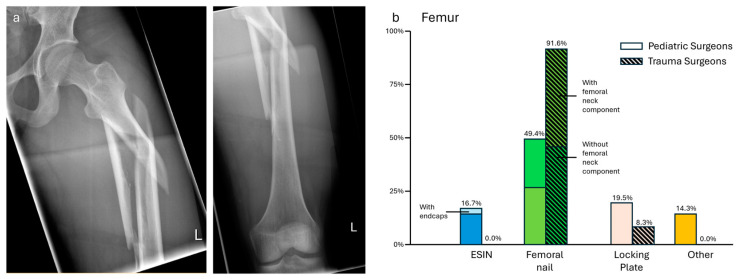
(a) X-ray of a multifragmentary femoral fracture (f, 17 y, 165 cm, 55 kg). (b) Descriptive data of the survey results, indicated in percentage.
